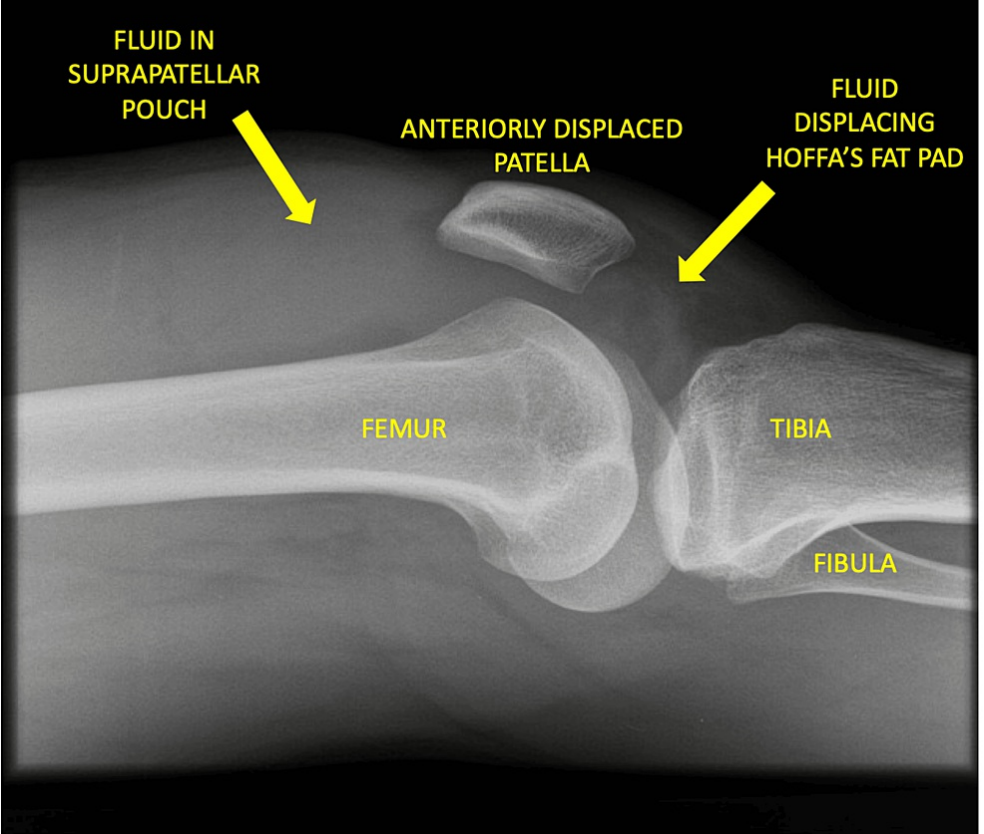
Plain lateral X-ray of the knee showing a joint effusion Credit: Image adapted from Radiopaedia [23]. Published under Creative Common License.
